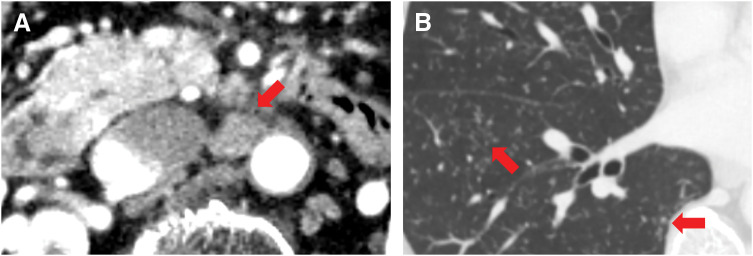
(A) CT image taken 1 year postoperatively showing enlargement of the para-aortic lymph nodes (red arrow). (B) CT taken 1 year postoperatively showing multiple grainy shadows in the lungs (red arrows).
